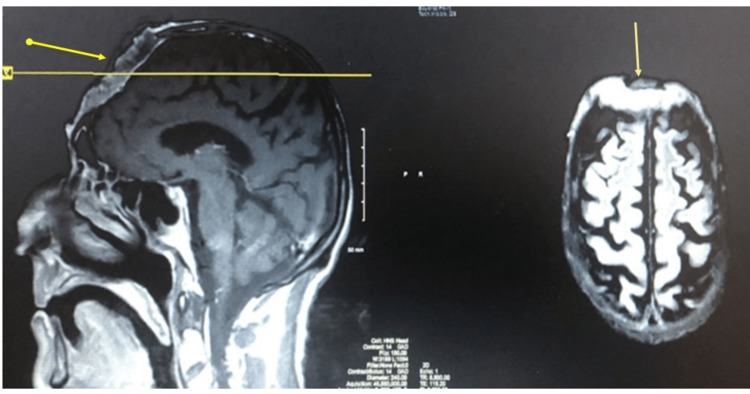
MRI skull and brain of the patient on presentation. The arrows show the eroding lesion through the skull into the dura. MRI: magnetic resonance imaging.
